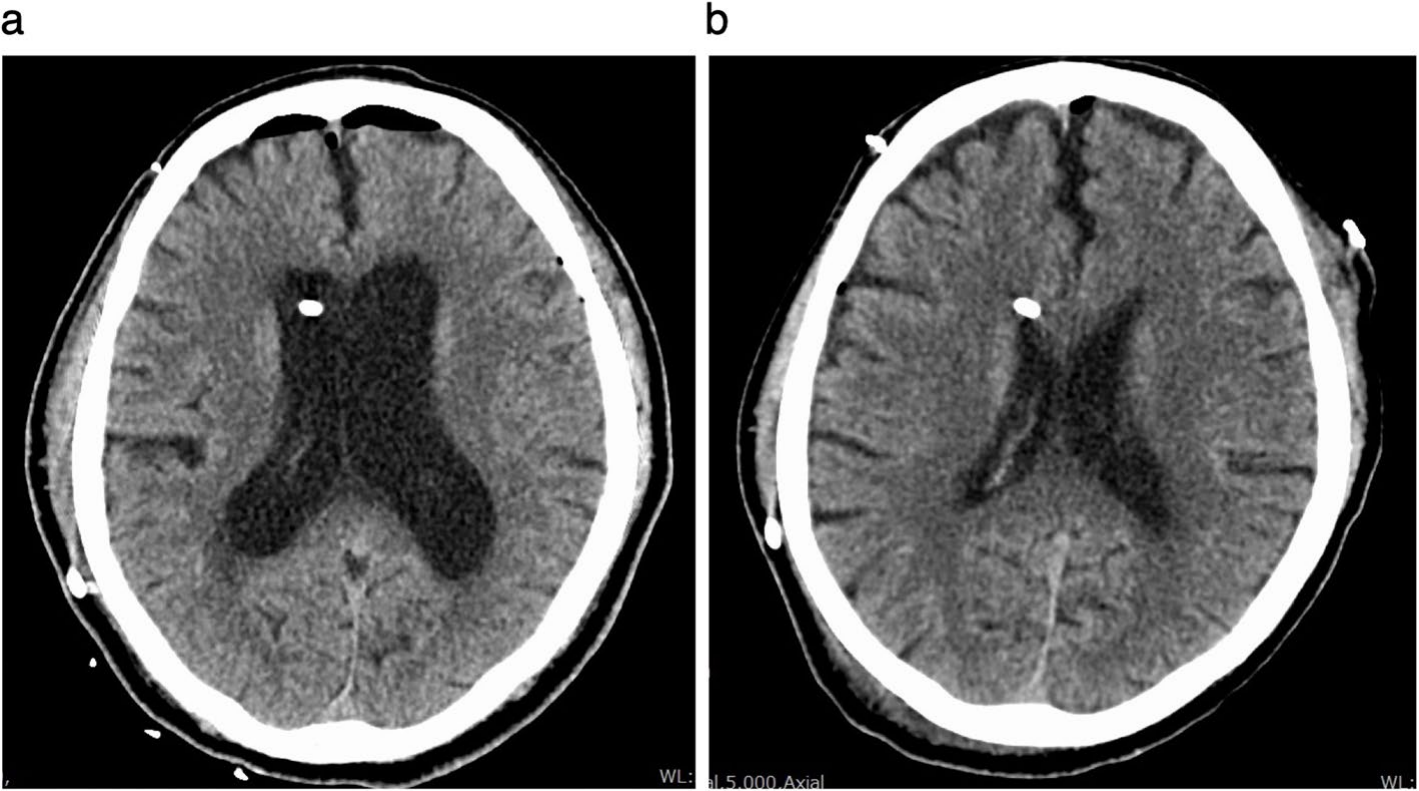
Head CT at just after surgery (a) and at the second postoperative day (b) after extubation
